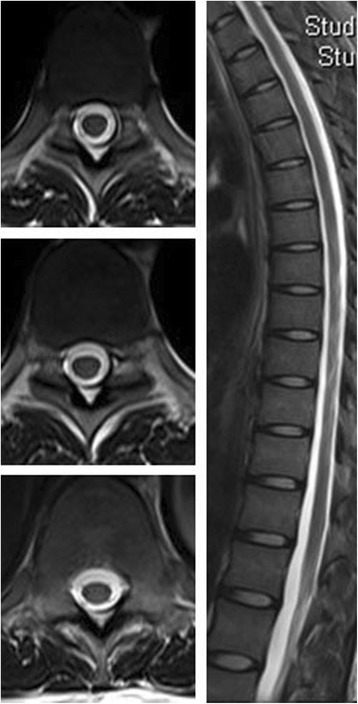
Follow-up thoracic spinal cord of MRI showed significant remission of the posterior columns’ signal alterations
